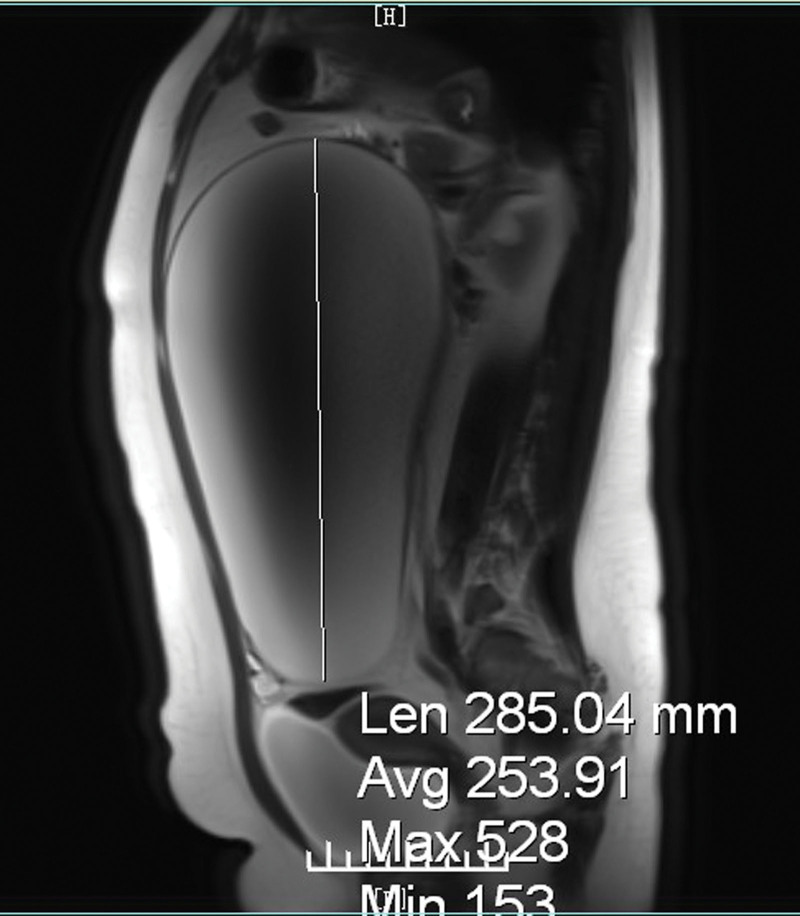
Magnetic resonance imaging of the abdomen and pelvis revealed a huge cystic mass in the adnexal torsion and a suspected cystadenoma.
